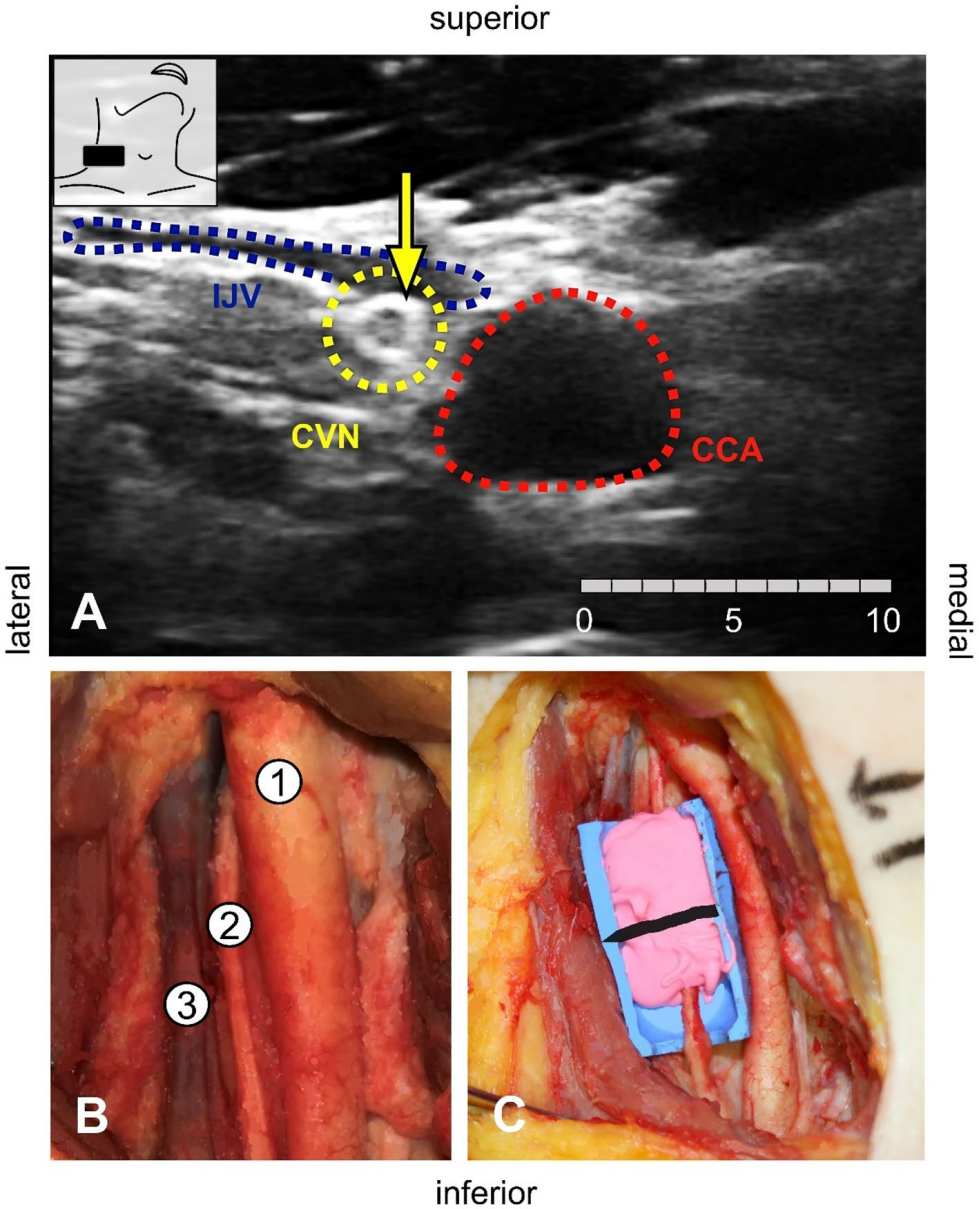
(A) The cervical vagus nerve (CVN), the internal jugular vein (IJV), and the common carotid artery (CCA) are shown using high-resolution ultrasound. The yellow arrow points out the nerve’s hyperechoic epineurium. The nerve’s cross-sectional area is measured within this structure (scale in mm). (B) Preparation of the common carotid artery (1), the cervical vagus nerve (2), and the internal jugular vein (3). Most often the nerve is found in between the great vessels. (C) Casting of the cervical vagus nerve with pink-colored dental molding silicon. The blue mold provides sufficient casting by separating the nerve from surrounding tissue and moisture. The black line marks the level of examination.
